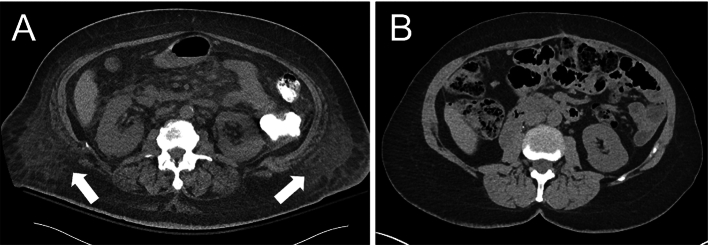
Comparison of patients without and with edema on abdominal CT. Edema is visually represented as heterogeneous regions within the adipose tissue (arrows, A). In contrast, adipose tissue is homogenous in a patient without edema (B)
